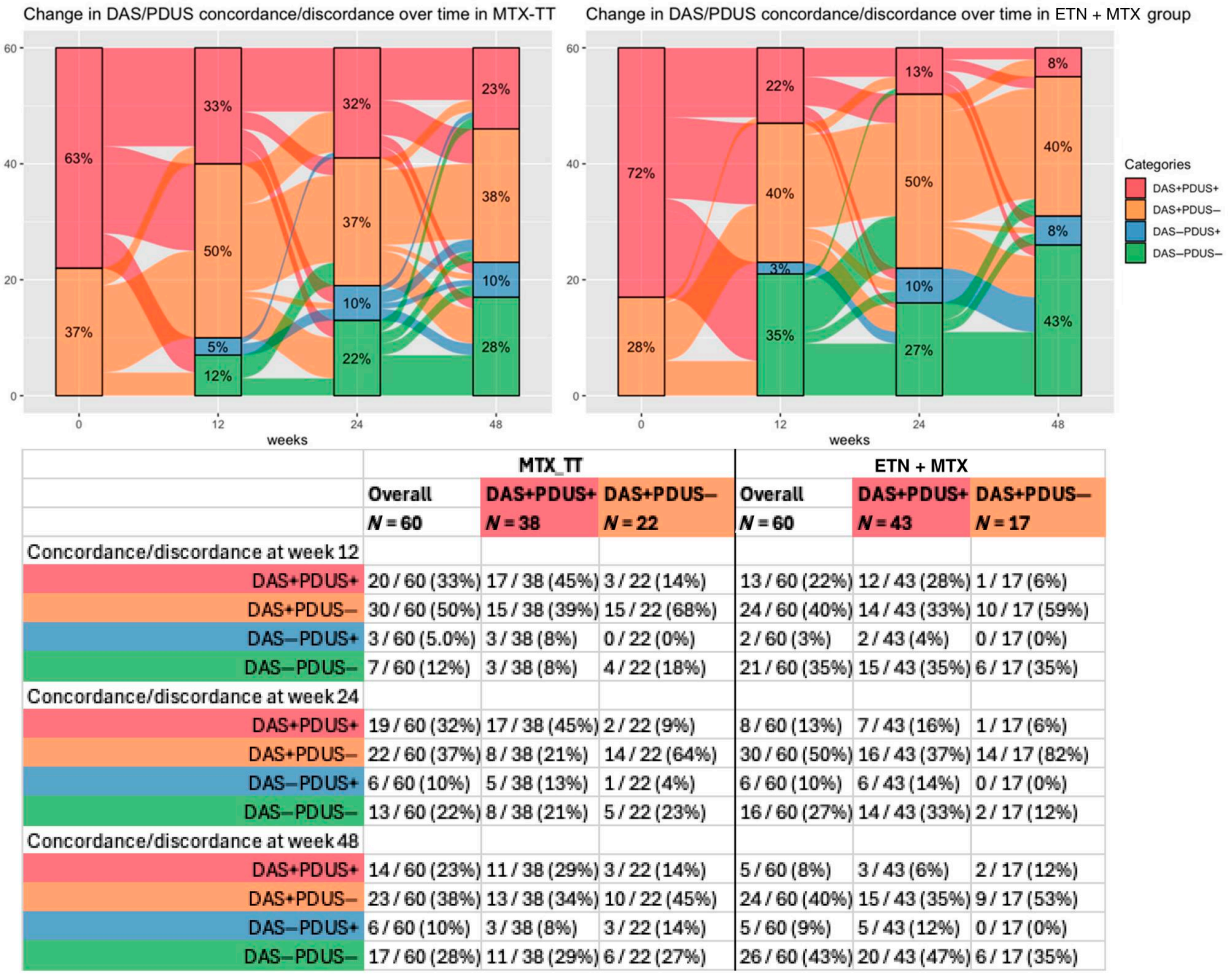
Longitudinal change in concordance/discordance states of DAS28-ESR and PDUS by treatment group. Left = MTX-TT group, Right = ETN+MTX group. DAS+PDUS+: DAS28-ESR > 2.6 and PDUS > 0; DAS+PDUS−: DAS28-ESR > 2.6 and PDUS = 0; DAS−PDUS+: DAS28-ESR ≤ 2.6 and PDUS > 0. Top image: Alluvial plot highlighting the transitions during the trial period. Lower table: week-wise transition states from baseline DAS+PDUS+ and DAS+PDUS− groups. PDUS: power Doppler joint synovitis; MTX-TT: MTX (treat-to-target); ETN + MTX: etanercept + MTX
